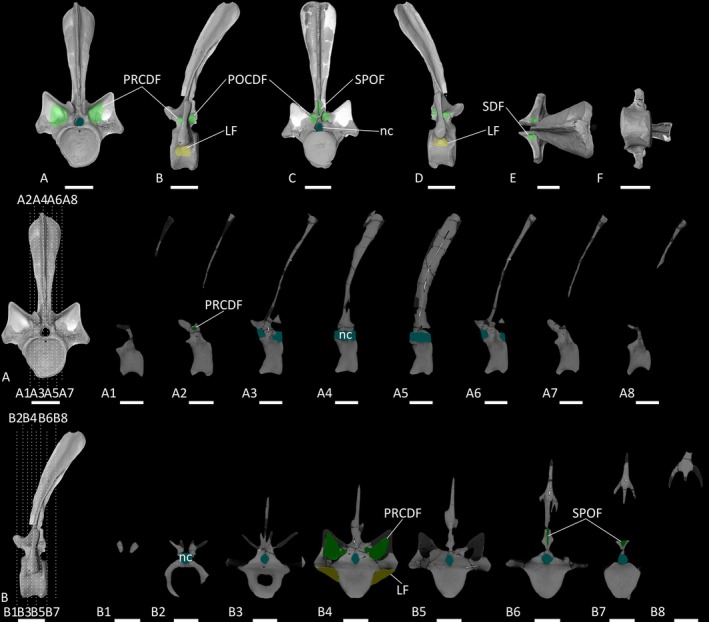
CT scan of *Dicraeosaurus sattleri* caudal vertebra 3 MB.R.3683. (A–F) 3D rendering (A) anterior view, (B) left lateral view, (C) posterior view, (D) right lateral view, (E) dorsal view, (F) ventral view; (A1–A8) CT scan slices in parasagittal cross‐section, (B1–B8) CT scan slices in transverse cross‐section. LF, lateral fossa; nc, neural canal; POCDF, postzygapophyseal centrodiapophyseal fossa; PRCDF, prezygapophyseal centrodiapophyseal fossa; SDF, spinodiapophyseal fossa; SPOF, spinopostzygapophyseal fossa. Key: Blue highlight, neural canal; green highlight, neural spine fossa; yellow highlight, centrum fossa. Scale bars = 100 mm.
